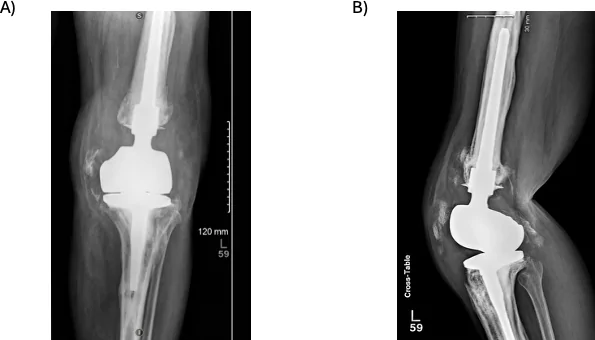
Preoperative radiographs. (A) Anteroposterior view demonstrating a well-aligned cemented hinged distal femoral endoprosthesis with no evidence of loosening, subsidence, or periprosthetic fracture. (B) Lateral view confirming stable positioning of the femoral and tibial components, intact cement mantle, and absence of implant migration or mechanical failure.
